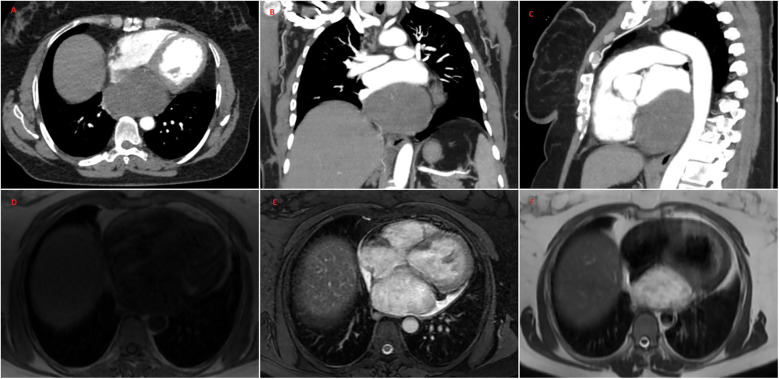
Thoracic CT angiography (A–C) and MRI (D–F) images of the patient. CT and MRI show a well-circumscribed pericardial mass measuring 10.1 cm × 8.1 cm × 5.2 cm located in the posterior mediastinum. CT and MRI demonstrated no evidence of the invasion of the inferior vena cava, left atrium, left ventricle and pulmonary veins. MRI showed that the mass was T1-hypointense (D) and T2-hyperintense (F) The mass was observed to have homogeneous signal intensity on T1 and T2-weighted images (D–F) The mass showed high signal intensity on T2-weighted fat suppression sequence (E) CT, computed tomography; MRI, magnetic resonance imaging.
